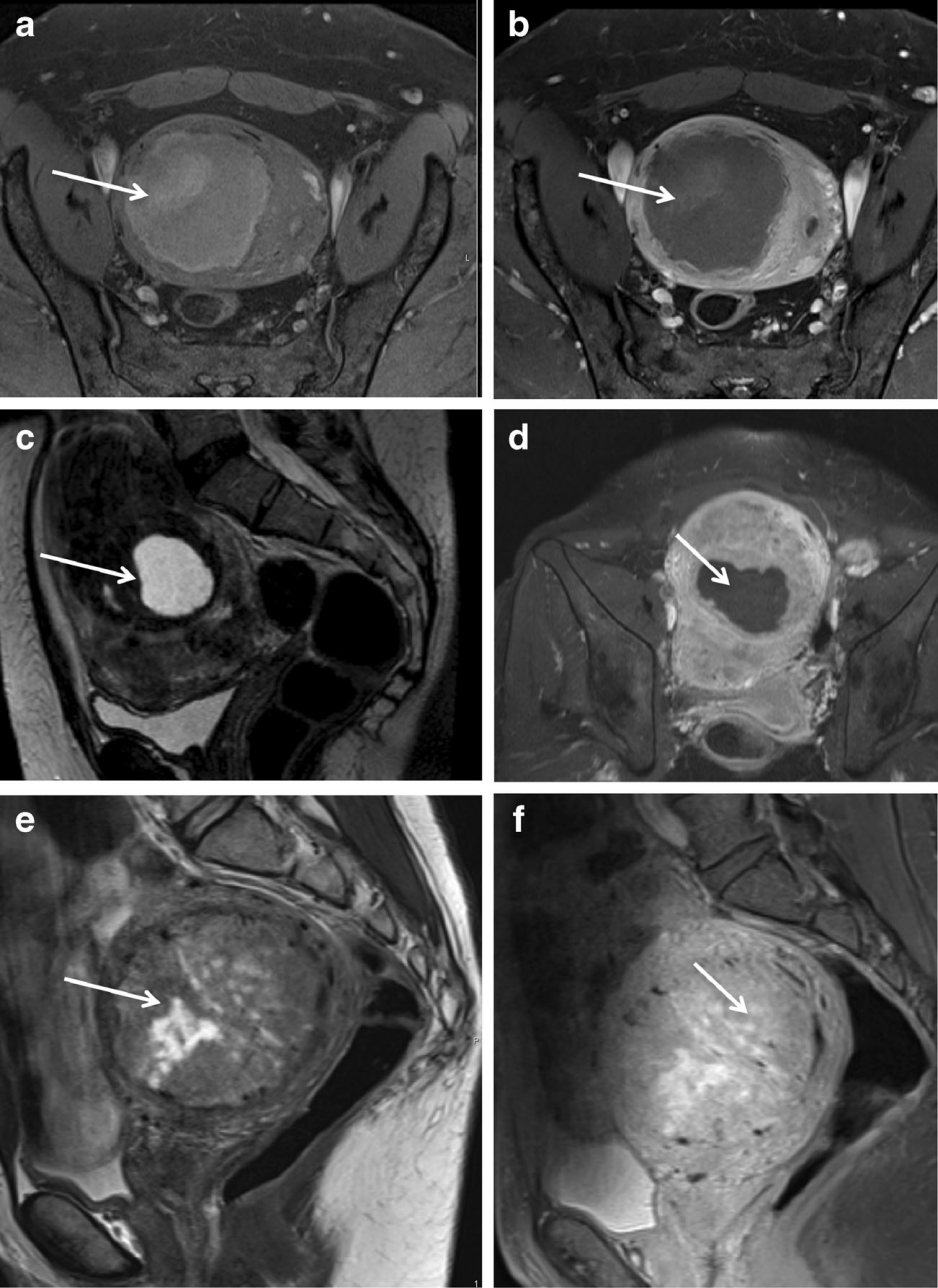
Different aspects of leiomyoma degeneration: Hemorrhagic degeneration after UFE. Axial T1-weighted fat saturated images, before (a) and after gadolinium administration (b) show a leiomyoma with increased signal on T1 and lack of enhancement, consistent with hemorrhagic degeneration. Cystic degeneration. Sagittal T2-weighted (c) and axial T1-weighted fat saturated contrast enhanced (d) images show a leiomyoma presenting a peripheral area with high T2 intensity signal and no enhancement, consistent with cystic degeneration (arrows). Myxoid degeneration. Sagittal T2-weighted (e) and T1-weighted fat saturated contrast enhanced (f) images show a leiomyoma presenting areas with high T2 intensity signal and enhancement after gadolinium administration (in contrast with the cystic degeneration), suggesting myxoid degeneration (arrows)
